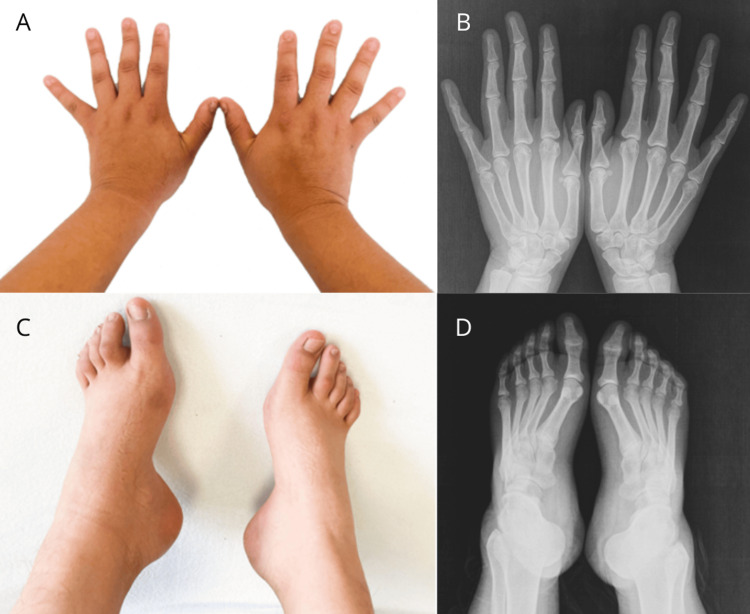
Bilateral cavus foot and camptodactyly with lateral deviation of the distal phalanges X-ray images confirming bilateral cavus foot and camptodactyly with lateral deviation of the distal phalanges of the middle fingers in both hands. (A-B) Hands in dorsopalmar view, showing camptodactyly with lateral deviation of the distal phalanges of both middle fingers. (C-D) Bilateral cavus feet, shown in dorsal view; radiographs obtained in anteroposterior (AP) view
